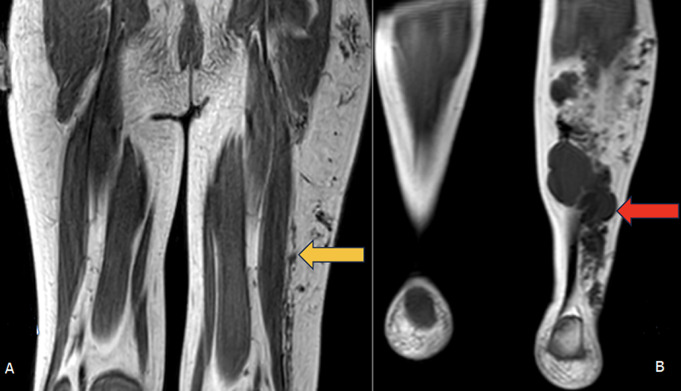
A) coronal T1-weighted MRI demonstrating diffuse hypertrophy of the left lower limb with hypointense vascular channels and phleboliths (yellow arrow); B) coronal T1-weighted MRI of the left lower limb showing multiple well-defined T1 hypointense lesions (red arrow) extending through fascial planes, consistent with venous malformation
